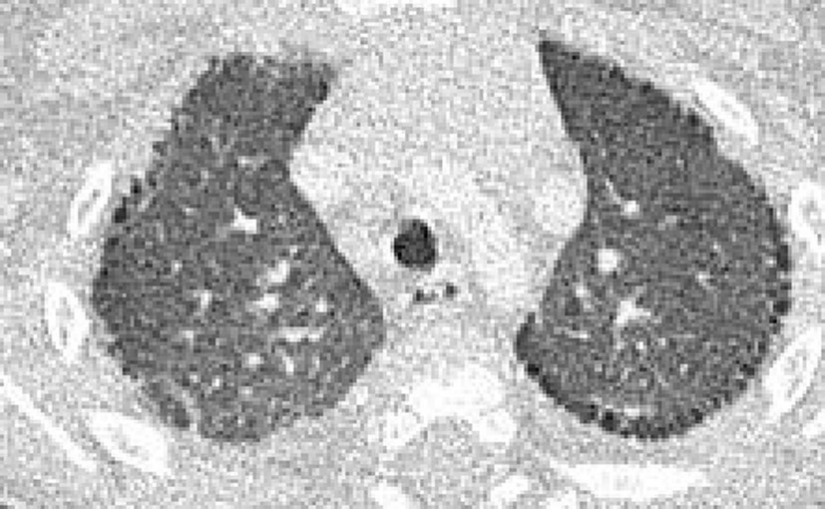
Alveolar growth abnormality due to trisomy 21. An infant with congenital heart disease. Preoperative CT unexpectedly uncovered small cysts seen extensively in the subpleural region and to a certain extent in the central zone of the lung
